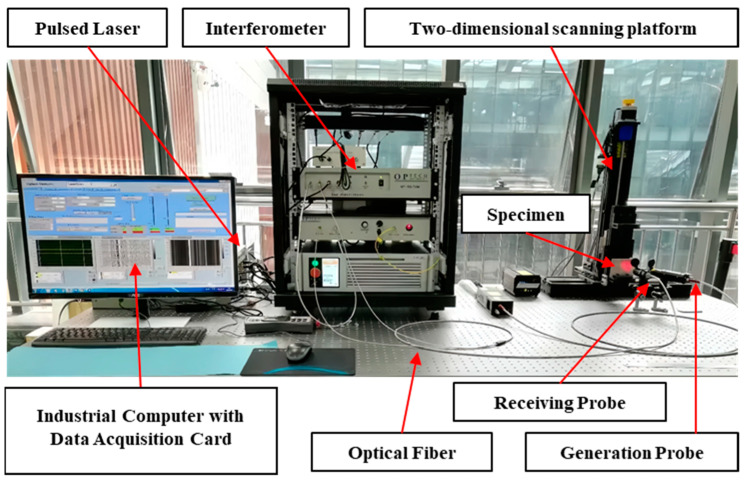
Laser ultrasonic testing system.
